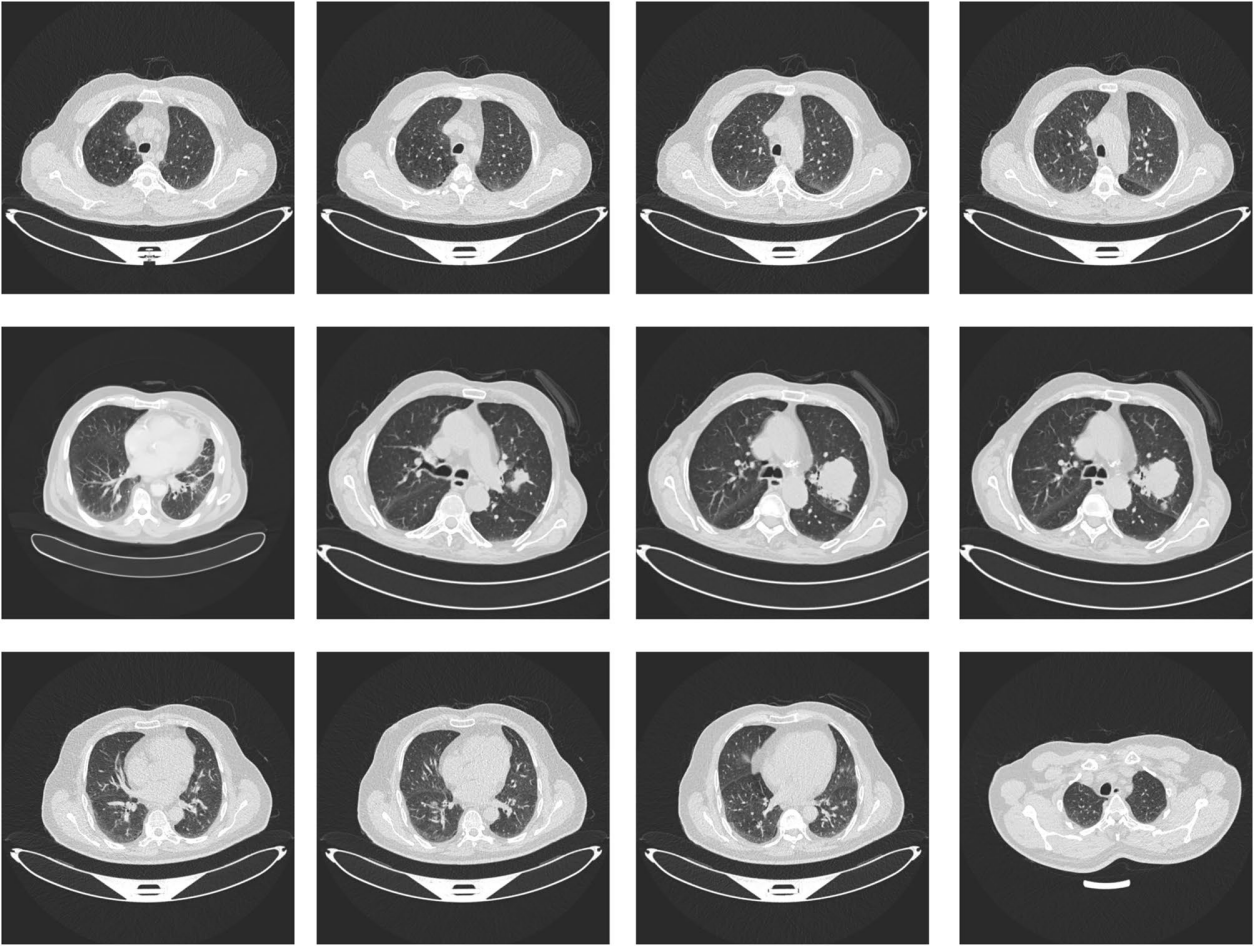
Representative CT images from the IQOTHNCCD Lung Cancer Dataset demonstrating Normal, Malignant, and Benign cases. Malignant cases show nodular densities, while benign cases exhibit non-cancerous lesions with well-defined borders.
